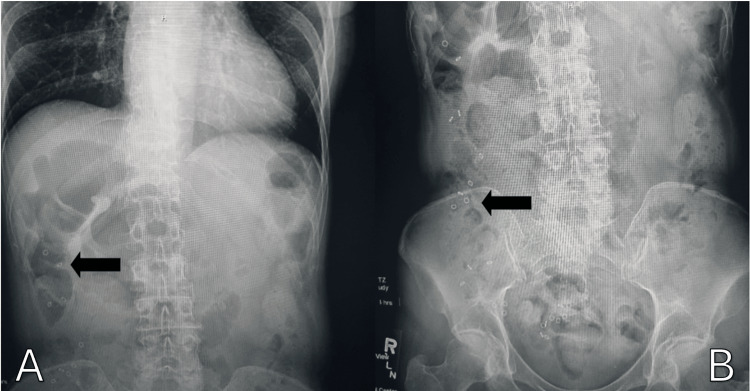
(A) AP X-ray with sitz marker. (B) AP X-ray with sitz marker: sitz marker study with marker residing mainly in the ascending colon after 48 hours AP: anteroposterior
